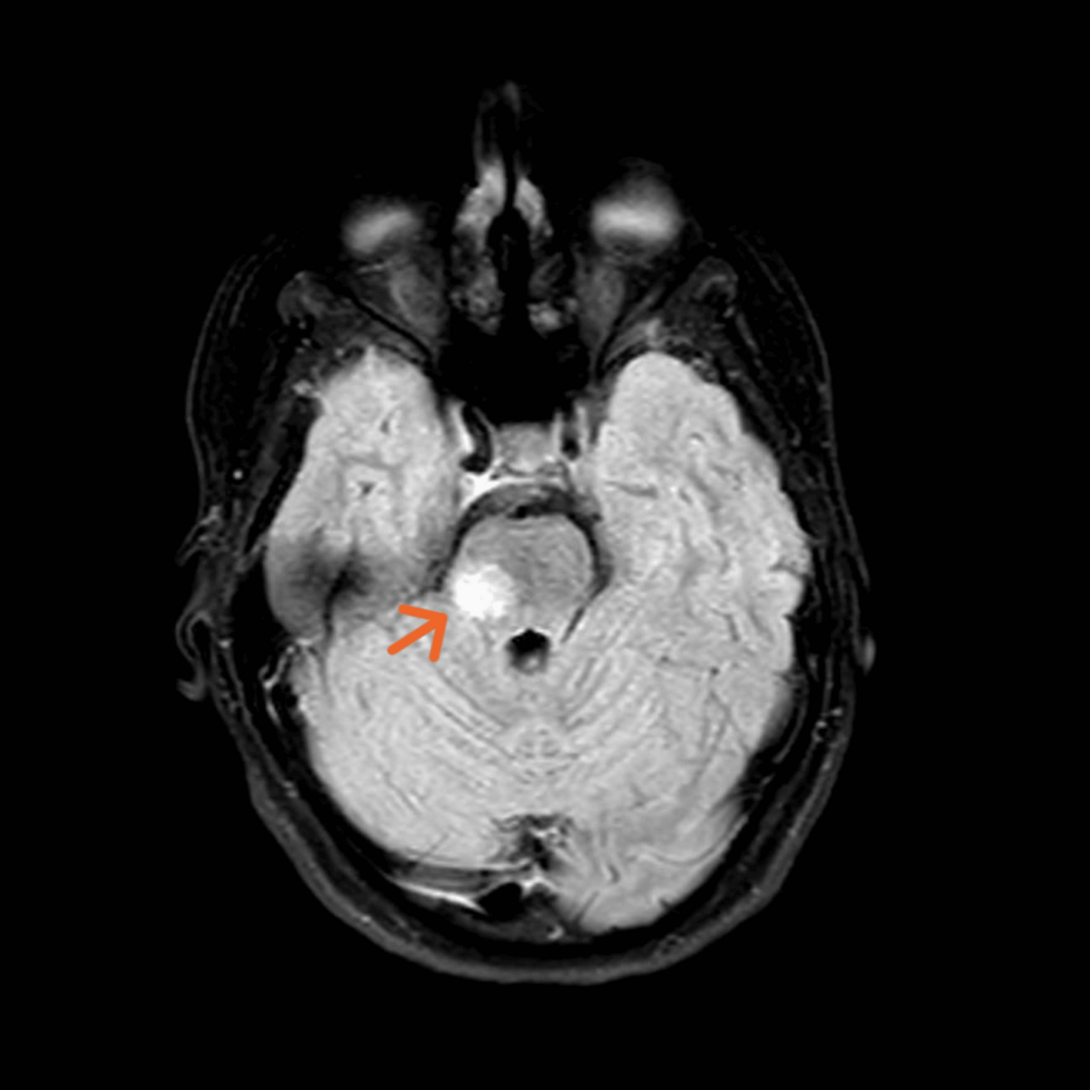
Axial FLAIR MRI of the head at the level of pons showing lesion on the right side of the pons and right middle cerebellar peduncle. FLAIR: fluid-attenuated inversion recovery
